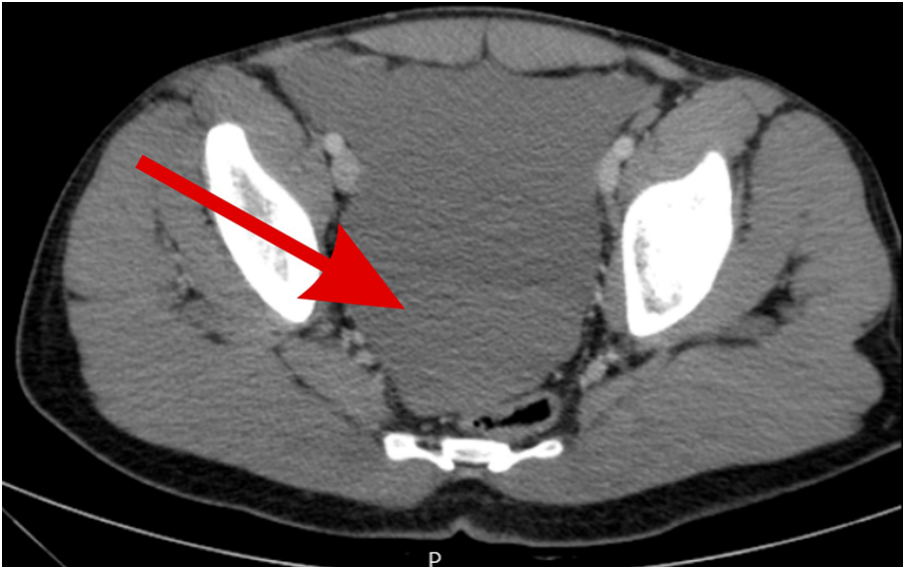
Pelvic CT scan showing free fluid in the pelvic area of fluid density indicating hemoperitoneum (red arrow).
